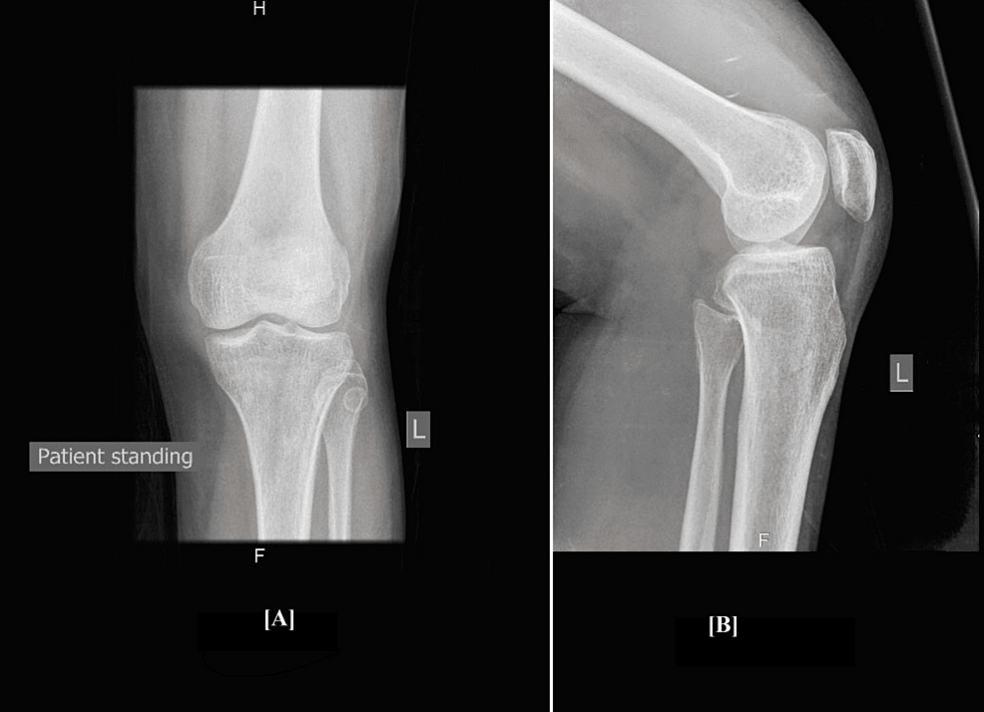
AP view (A) and lateral view (B) x-ray of the knee. The x-ray was unremarkable. AP: anteroposterior
